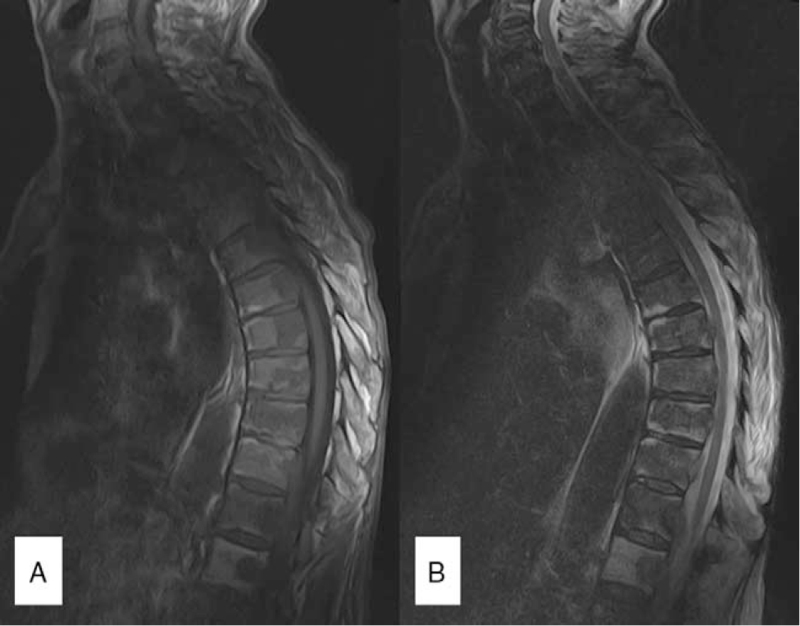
Thoracic magnetic resonance imaging scan – T1 (A) and T2-weighted (B) sagittal sections - thoracic vertebral bodies showing structural, shape alterations, and diffuse signal alterations (T1 and T2 hyposignal). Some of the vertebrae exhibit contrast-enhancement as well as thickening and contrast enhancement of their corresponding leptomeninges.
